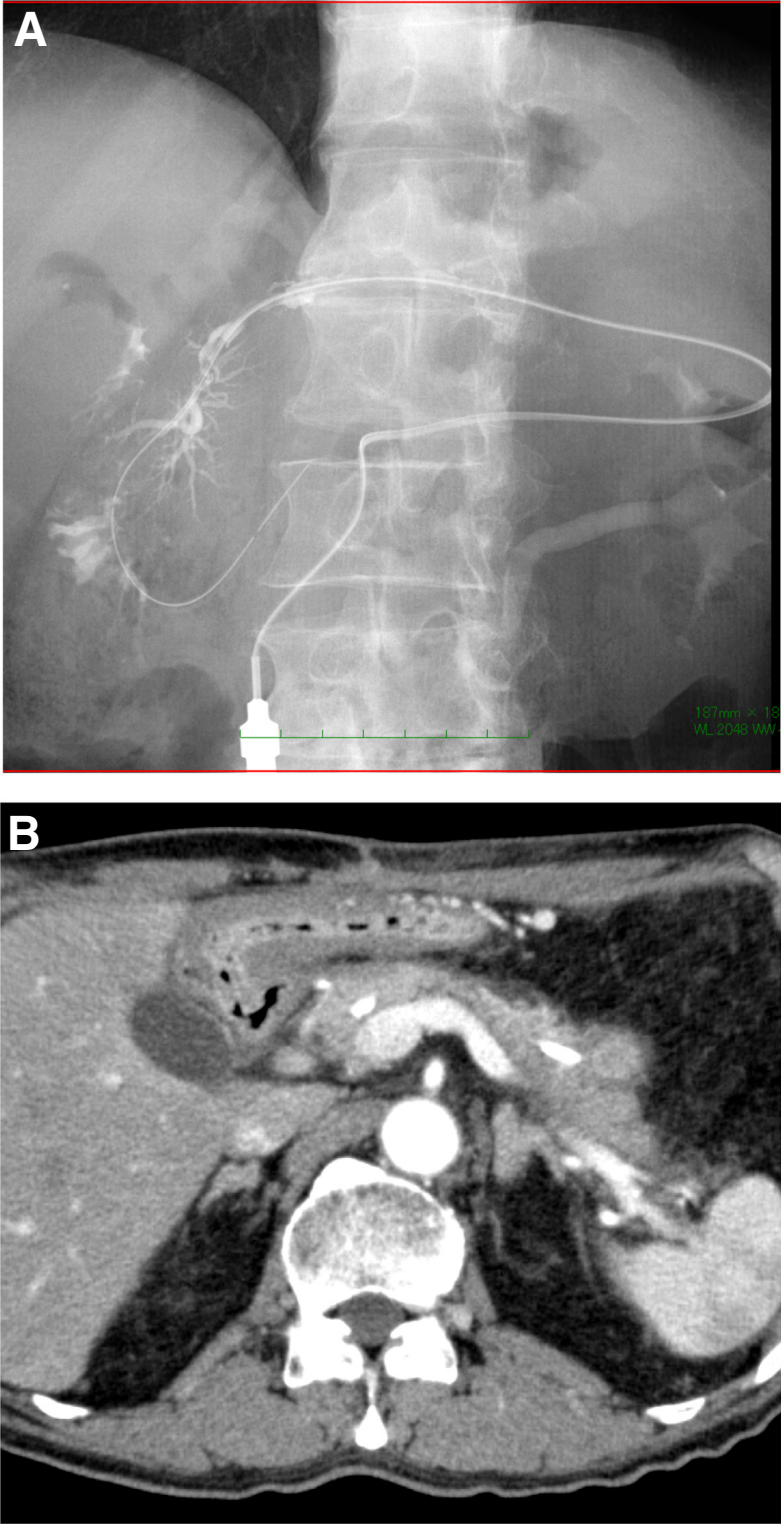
Disappearance of pancreatic pseudocyst. a The pancreatic pseudocyst was seen on pancreatography through the pancreatic drainage tube. b Enhanced CT showed that the pancreatic pseudocyst had disappeared 4 weeks after the second operation
